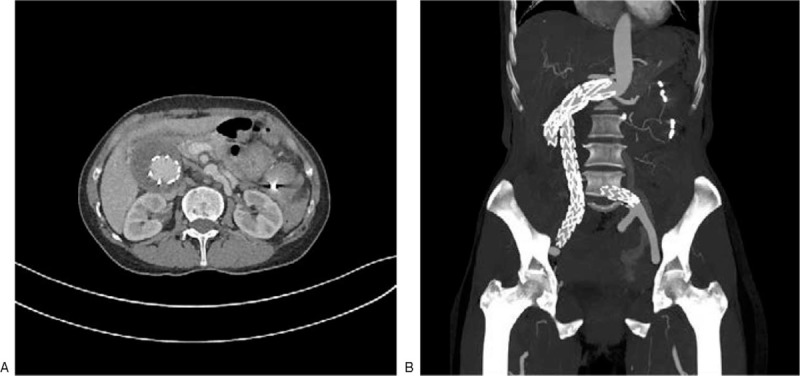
(A) Postoperative computed tomography (CT) reexamination showed that the pseudoaneurysm was not developed. (B) Postoperative aortic computed tomographic angiography (CTA) showing that the pseudoaneurysm was not developed.
